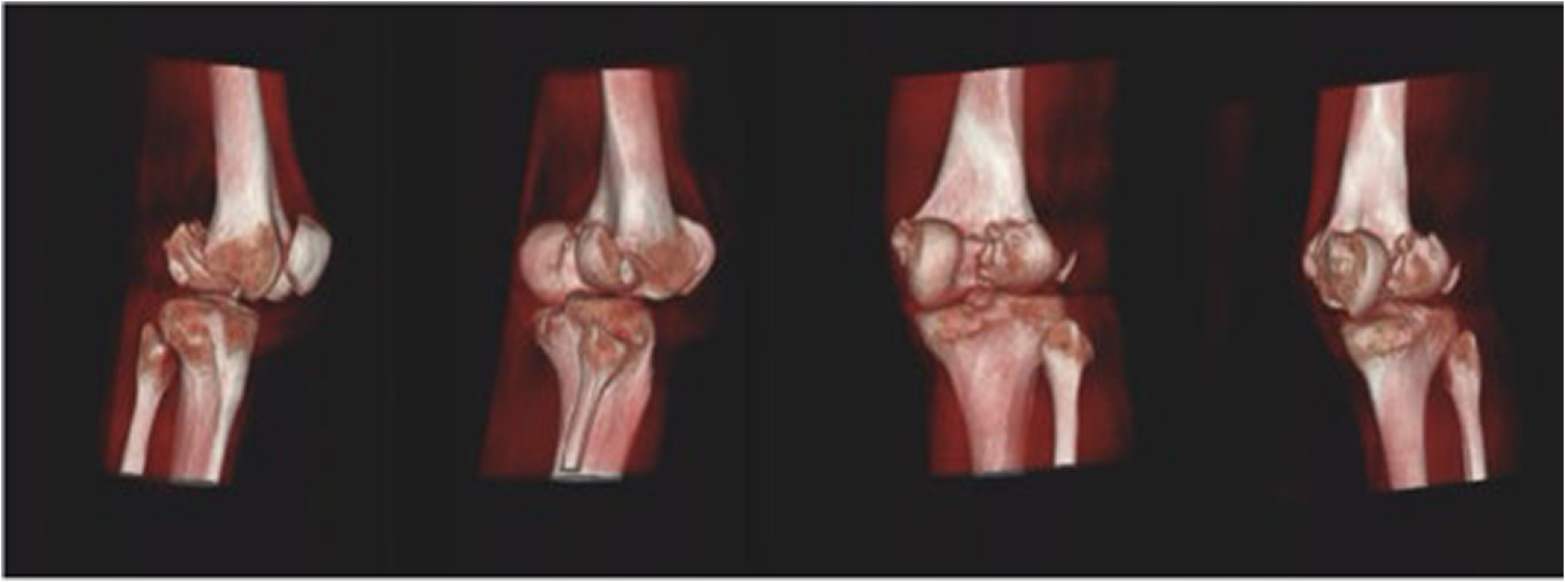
Medial and lateral femoral condyles fractures in the 3D‐CT‐scan.
